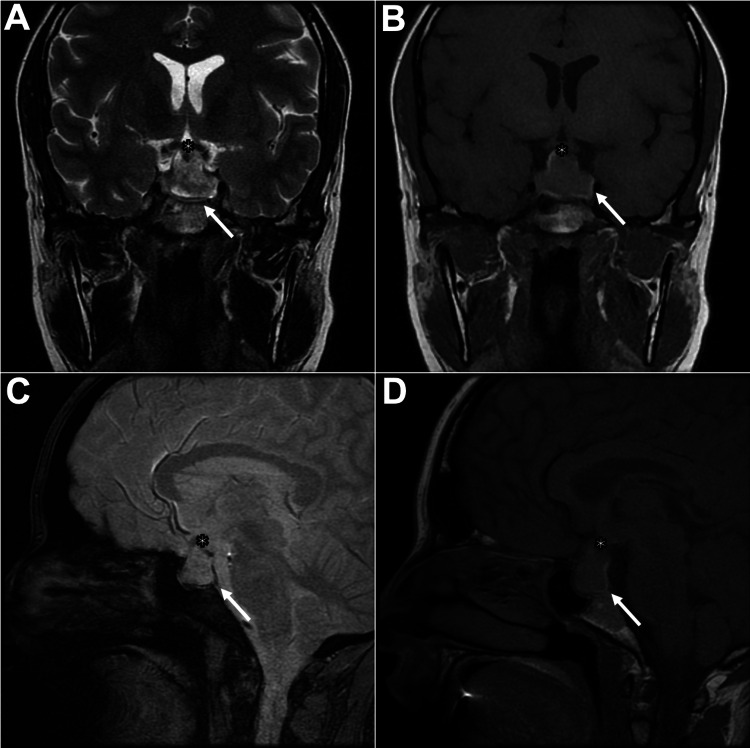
MRI on postpartum day 3 demonstrating hemorrhage within a pituitary macroadenoma, confirming apoplexy Coronal T2-weighted (A), coronal T1-weighted (B), sagittal T2*-weighted (C), and sagittal T1-weighted (D) images demonstrate interval growth of the pituitary lesion (23×25×13.5 mm) with marked suprasellar extension, contacting and displacing the optic chiasm superiorly (asterisk). The lesion also shows slight parasellar extension, abutting the medial wall of the cavernous sinuses. Increased internal heterogeneity is observed, with a peripheral rim that is hyperintense on T1-weighted (B, D) and hypointense on T2 (A) and T2*-weighted (C) images, consistent with acute hemorrhagic products (arrow). Findings are compatible with pituitary apoplexy.
